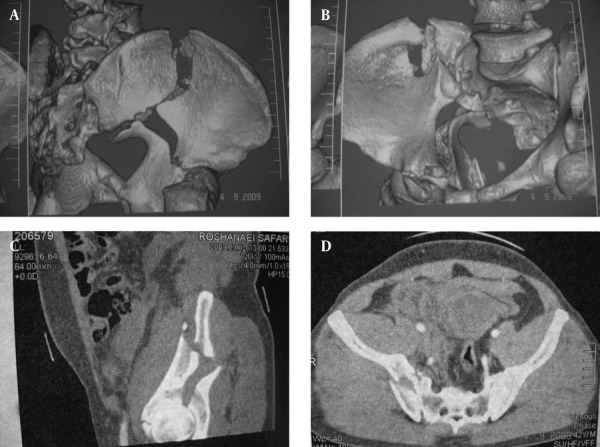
Preoperative CT Scans. A and B) Fracture of the iliac wing and fracture line in the weight bearing area of the SI joint is shown in the 3-D CTscan. Also note that the greater sciatic notch is separated without involvement of the posterior column; C and D) Sagittal and transverse views indicate the fracture of the iliac wing, superior articular surface and SI joint involvement.
